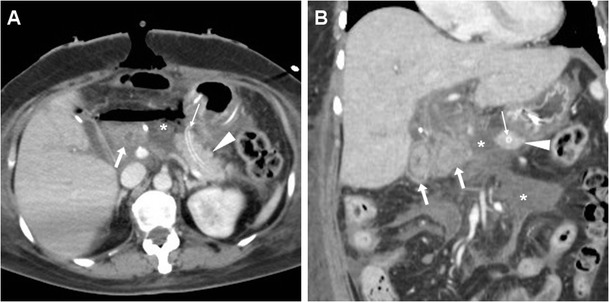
a, b Dehiscence of the PJS with peritonitis in a 59-year-old woman with shock, sepsis and peritonitis 48 h after PD performed for a benign tumour of the Vaterian ampulla. CT showed discontinuity between the PR (*arrowheads*) with MPD stent (*thin arrows*) and MJL (*arrows*), filled by a large air-fluid collection (*) extending in the mesentery. Emergency relaparotomy was performed, with creation of a gastro-pancreatic anastomosis
